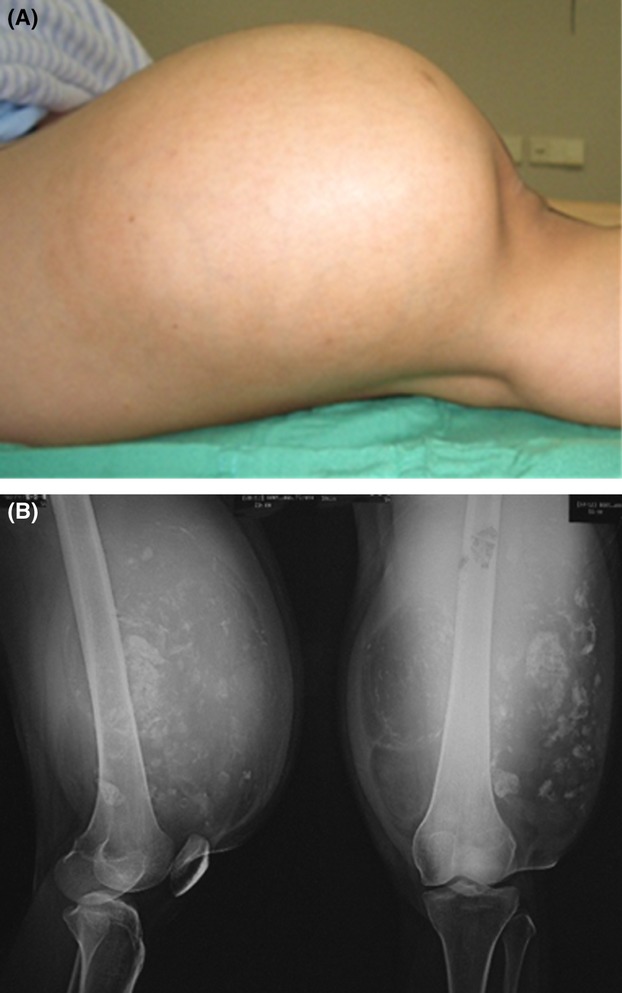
(A) Photograph showing a huge lump in the anterior part of the right thigh. (B) Radiographs revealed a bulged soft tissue mass in anterior compartment of right lower thigh showing predominantly radiolucent density with multiple chondroid matrix of calcification. Bone structure is still intact. (Reprint with permission from Reference.^25^)
